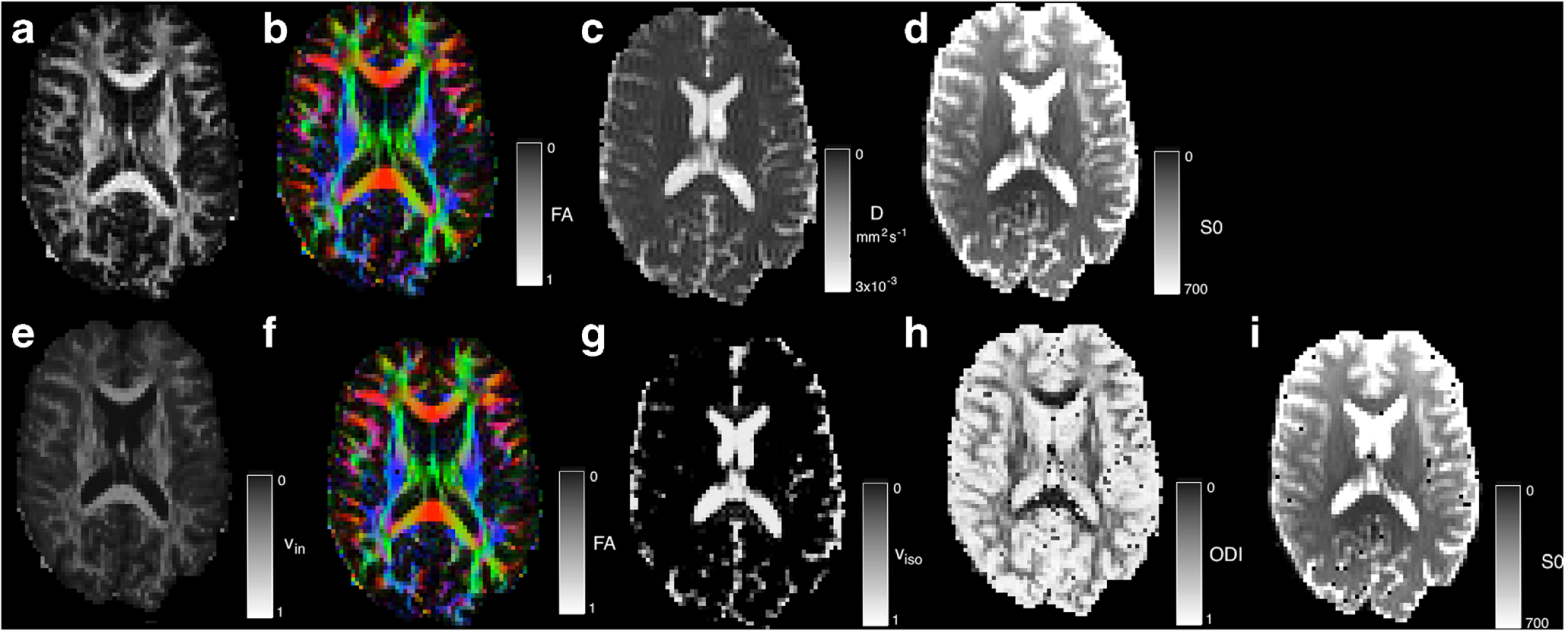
DWI parameter map comparison of DTI and NODDI parameters. a–d) represent DTI parameter estimates: a FA b colour-coded FA (*red* = ML, *green* = AP, *blue* = SI), c) the MD and d) S0. e–i) NODDI fitted parameters for e the intra-axonal volume fraction *v*
